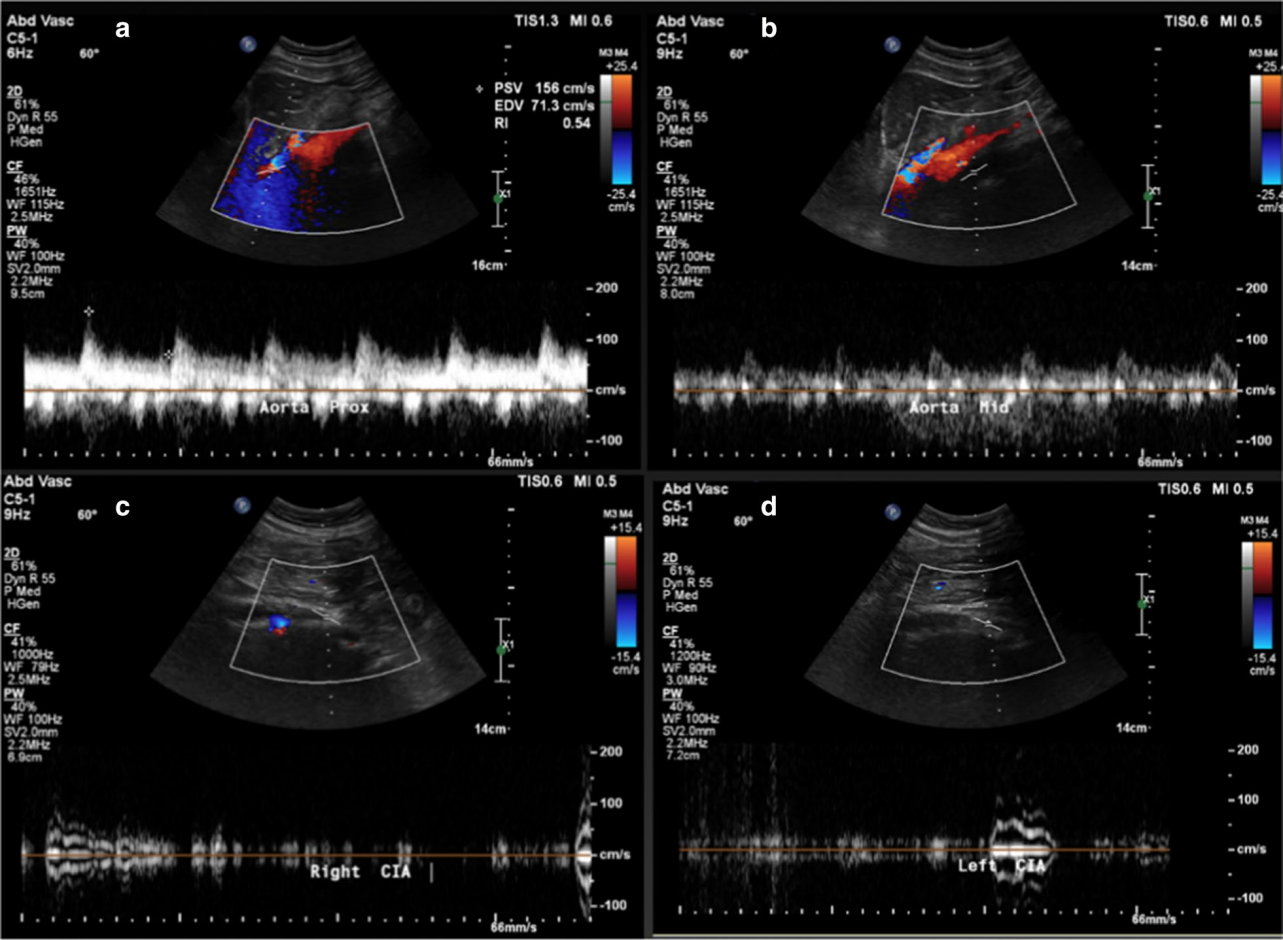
Ultrasound Doppler imaging of patient’s aorta and iliac arteries (a) proximal aorta, (b) mid aorta, (c) left common iliac artery, and (d) right common iliac artery
